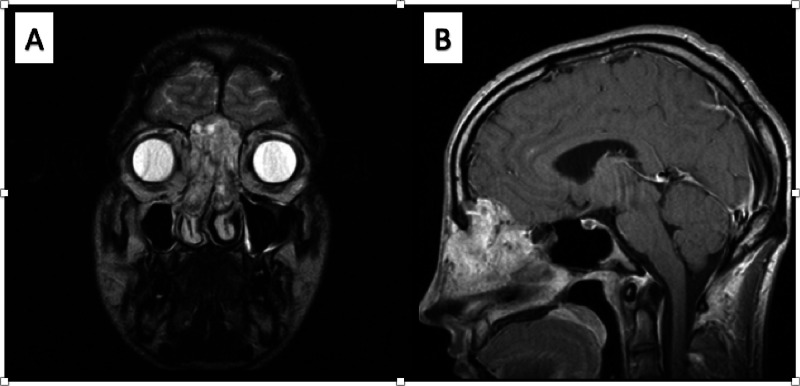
MRI imaging (A) Coronal MRI and (B) sagittal MRI revealing a 5.1 cm × 3.3 cm × 3.4 cm enhancing soft tissue mass centered in the ethmoid sinuses with invasion through the ethmoid plate and abutment of the bilateral frontal lobes. No gross invasion of frontal lobe parenchyma or dura identified.
